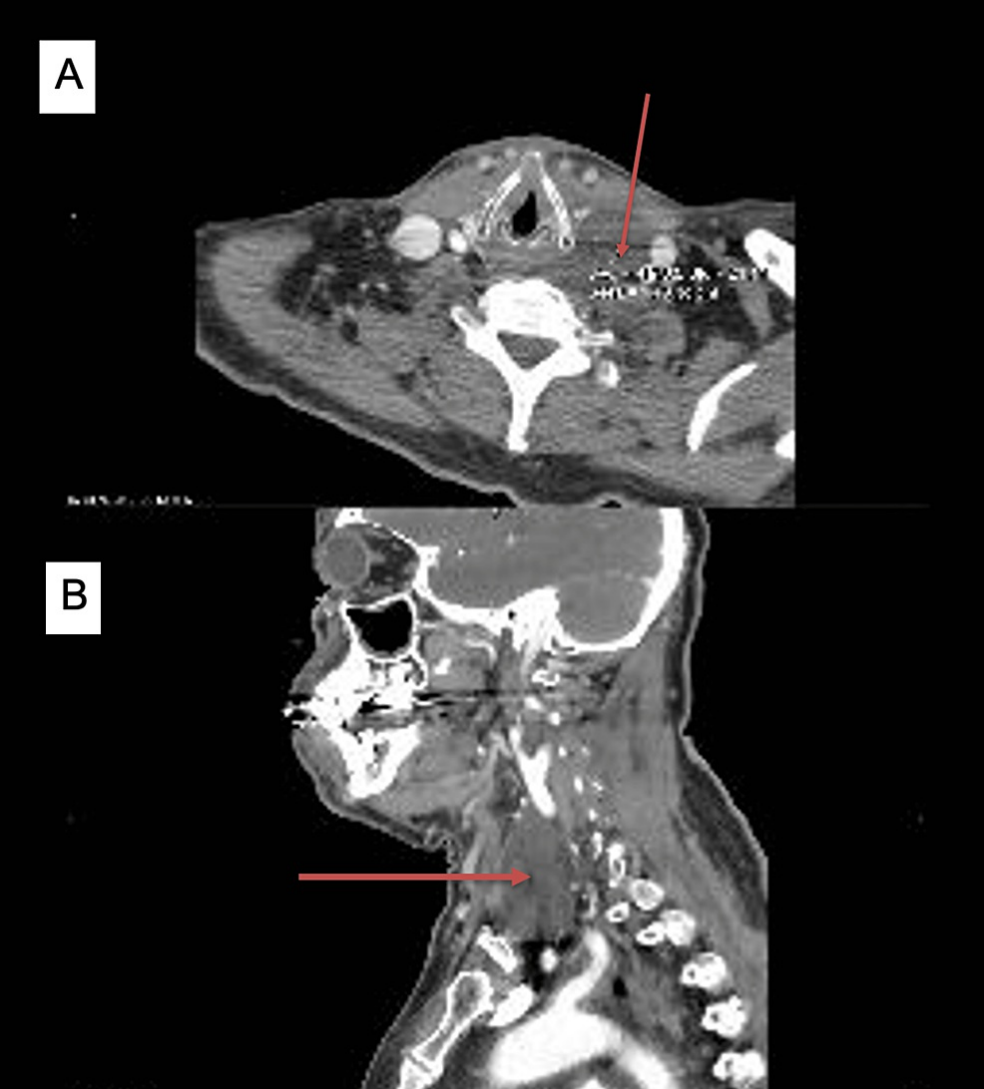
CT cervical soft tissue showing 5 cm L periesophageal/supraclavicular base fluid collection most consistent with lymphocele versus foregut duplication cyst. A: transverse section; B: sagittal section. CT = computed tomography
